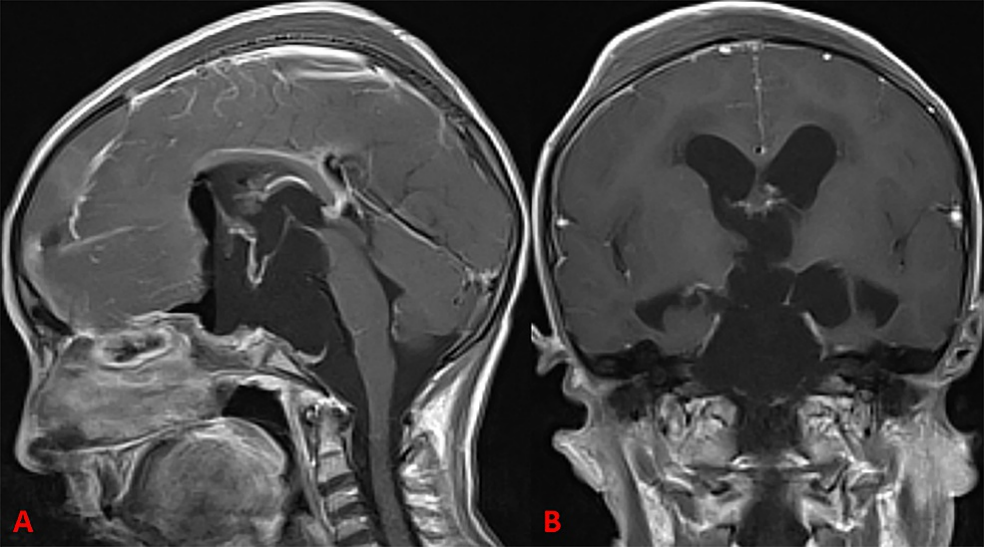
Immediate postoperative MRI Sagittal (A) and coronal (B) postcontrast T1-weighted images showing complete evacuation of cystic contents, decompression of neurovascular elements, and no obvious solid tumor component.
